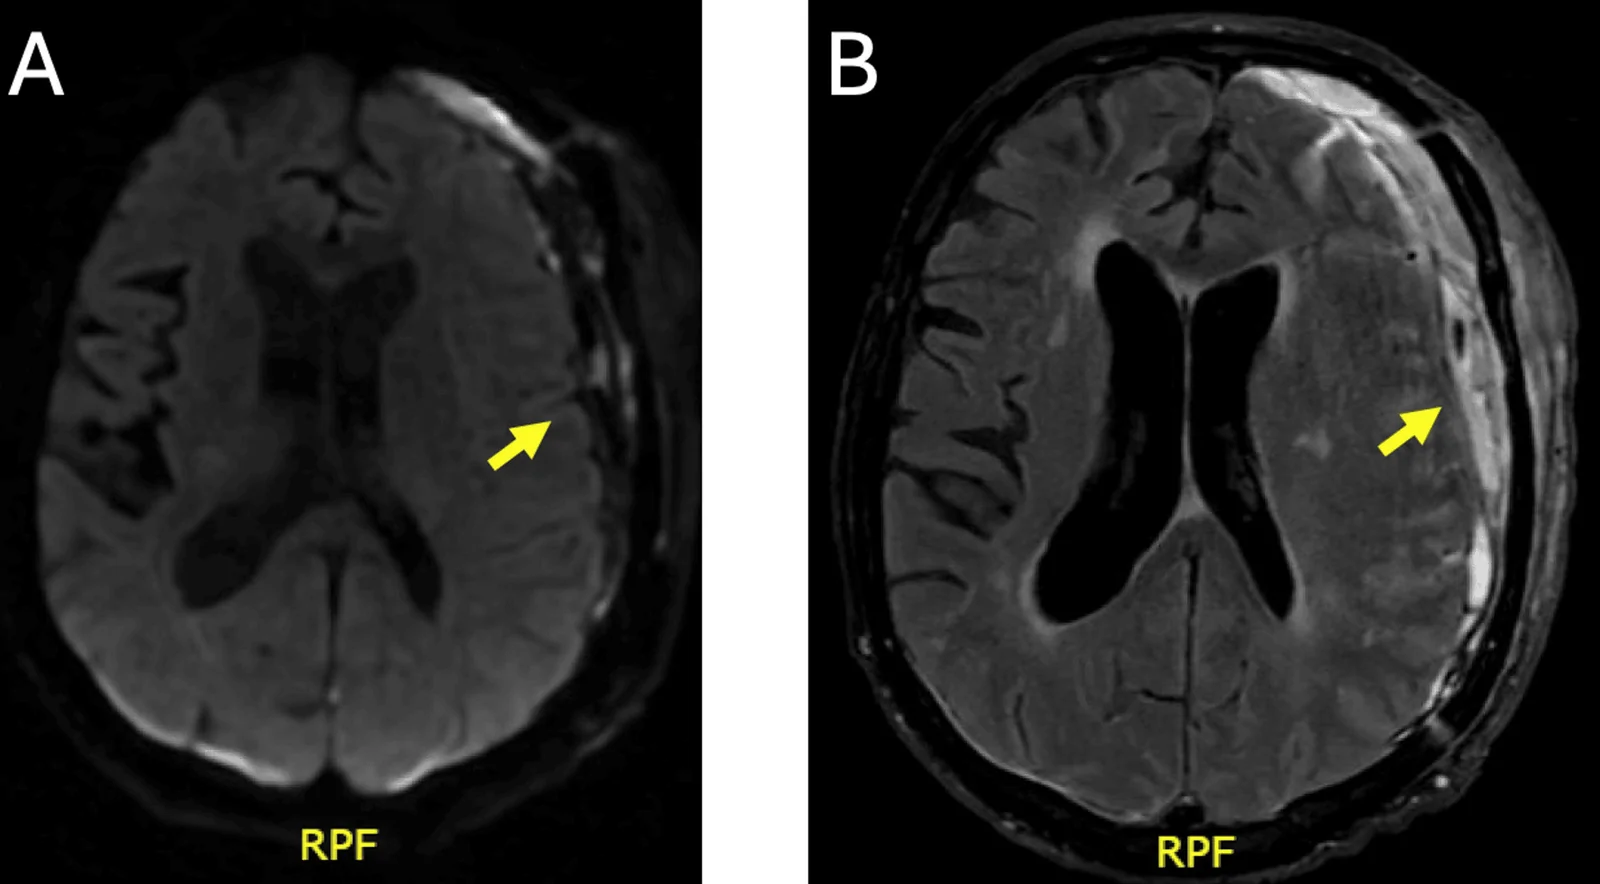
MRI of the brain demonstrating left-sided subdural empyema (A) Axial DWI sequence demonstrating marked diffusion restriction within the left subdural collection (yellow arrow). (B) Axial T2-weighted FLAIR image demonstrating extensive left hemispheric subdural fluid collection (yellow arrow) with adjacent edema and mass effect. DWI: diffusion-weighted imaging, FLAIR: fluid-attenuated inversion recovery, MRI: magnetic resonance imaging
